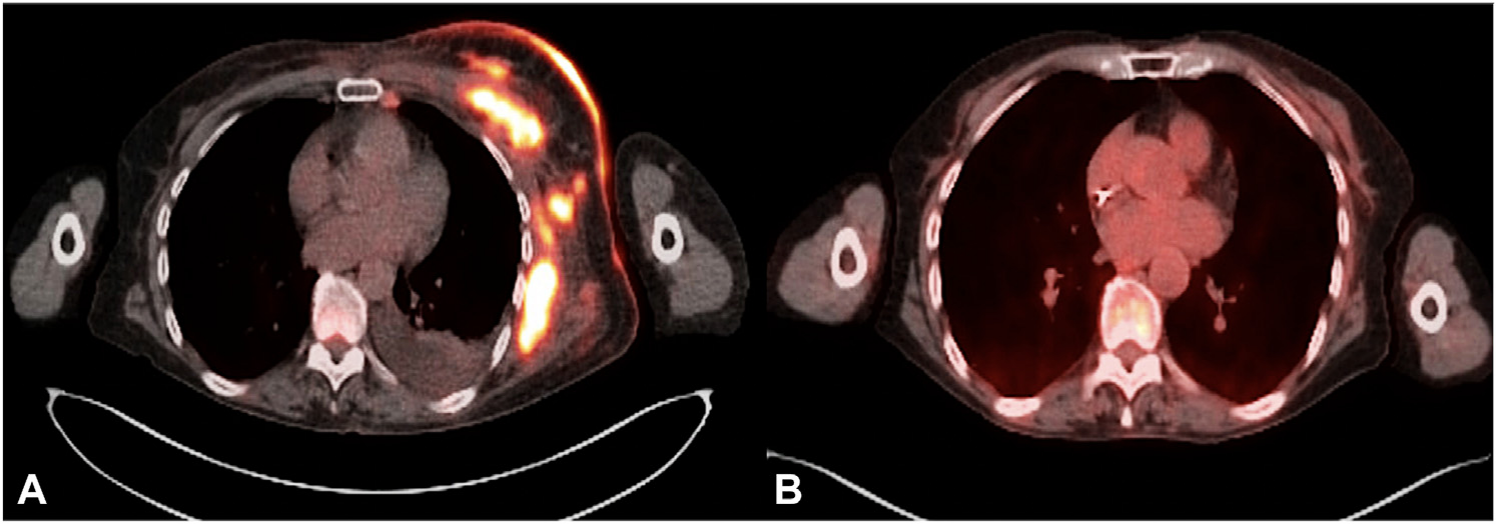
Positron emission tomography/computed tomography scan demonstrating increased fluorine-18 flurodeoxyglucose uptake (*red*) in the *left* breast and axilla (A) before treatment in comparison to the *left* breast and axilla (B) following 6 rounds of brentuximab vedotin and cyclophosphamide, doxorubicin, and prednisone chemotherapy.
